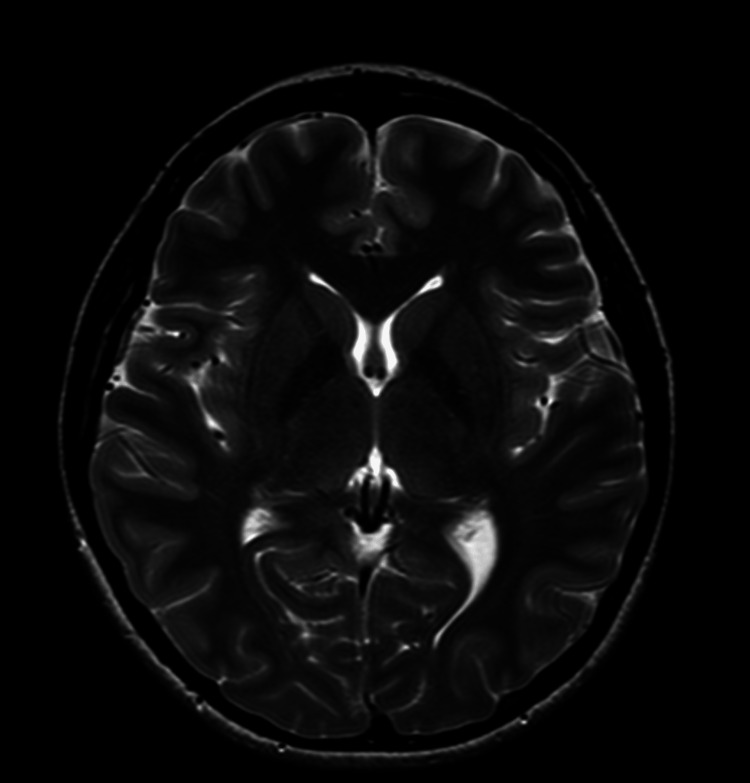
Non-contrast MRI-3T of the brain showing normal brain with no evidence of structural disease.
